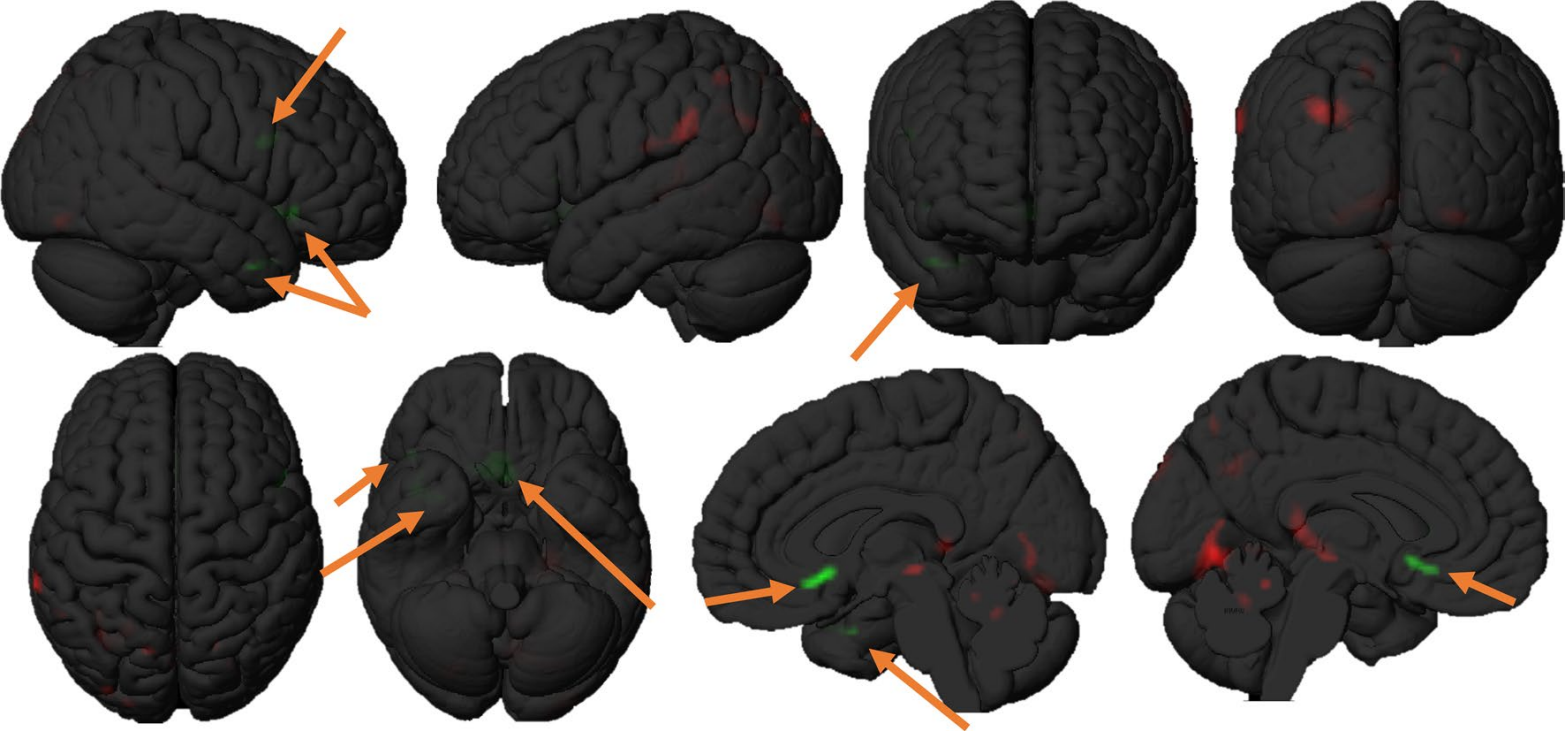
Regional [^18 ^F]-FDG PET hypometabolism in bvFTD PPA patients compared to AD PPA patients. Regions of significant differences in hypometabolism between bvFTD PPA patients and AD PPA patients were analysed using conjunction analysis. Results shown at *P* < 0.001. AD = Alzheimer’s disease; bvFTD = Behavioral variant frontotemporal dementia; PPA: primary progressive aphasia. It can be appreciated that in bvFTD PPA patients, a significantly lower metabolism was observed in the bilateral olfactory cortex, right temporal pole and right superior frontal gyrus (green clusters, indicated with gold arrows) compared to the metabolism observed in AD PPA patients. In AD PPA patients, on the other hand, a significantly lower metabolism was observed in the bilateral parahippocampal gyrus, bilateral lingual gyrus, left posterior cingulate cortex, left supramarginal gyrus, left superior parietal lobule. Additionally, a significantly lower metabolism was observed in AD PPA patients central in the midbrain, located in the region of the mamillotegmental tract
